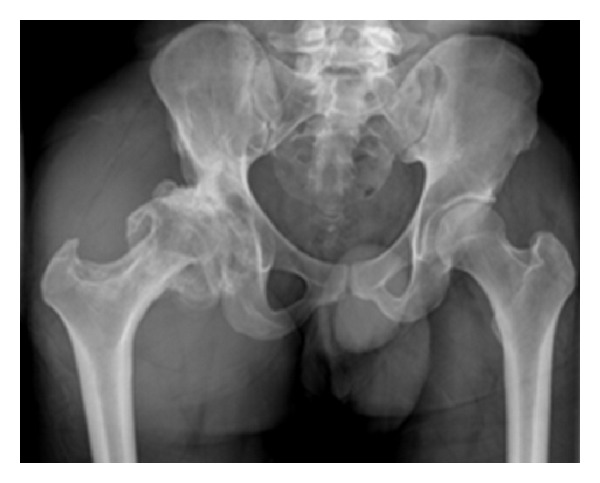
The preoperative radiographic demonstrates lateralization and subluxation of the right hip, osteoarthritis of hip joint, and excessive offset deformity with a longer neck of femur.
